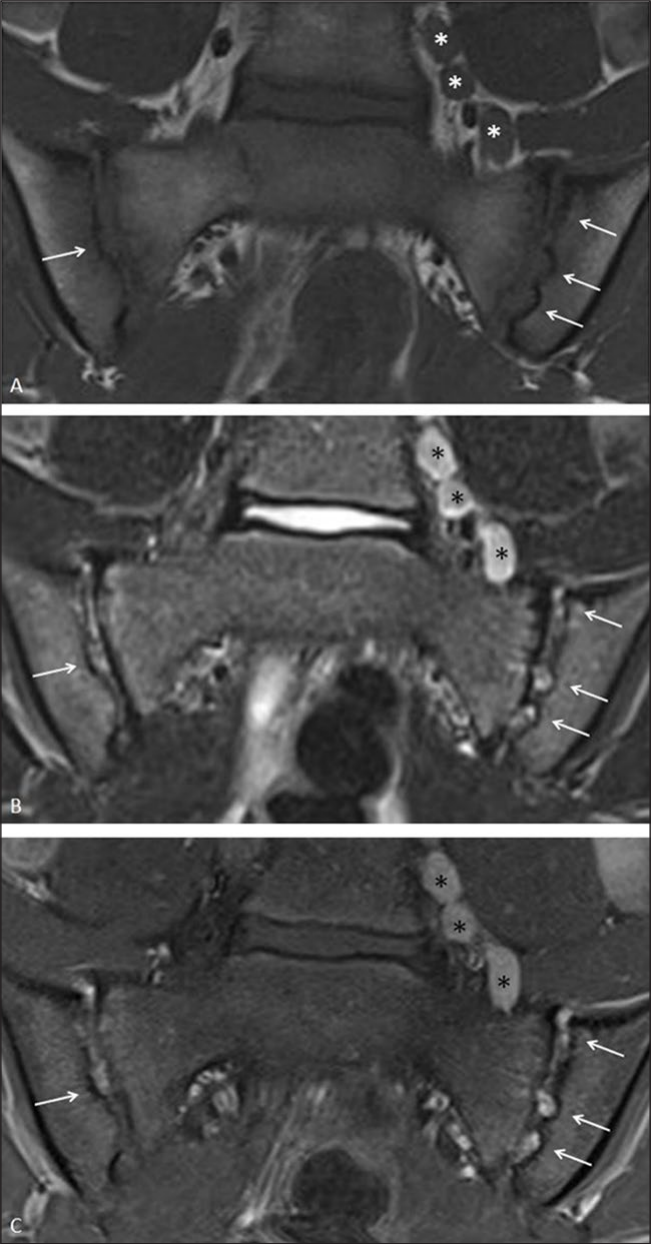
Active and structural sacroiliitis in a 17-year-old boy with JSpA. a. Semicoronal T1-weighted image show extensive erosions of the SI joints (arrows). b. Semicoronal STIR image shows high signal in the joint space and within the erosions (arrows). No surrounding bone marrow edema is visible. c. Contrast-enhanced fat-saturated T1-weighted image shows synovial enhancement in the erosions and the joint space. Note also the enlarged lymph nodes along the iliac vessels (asterisk).
